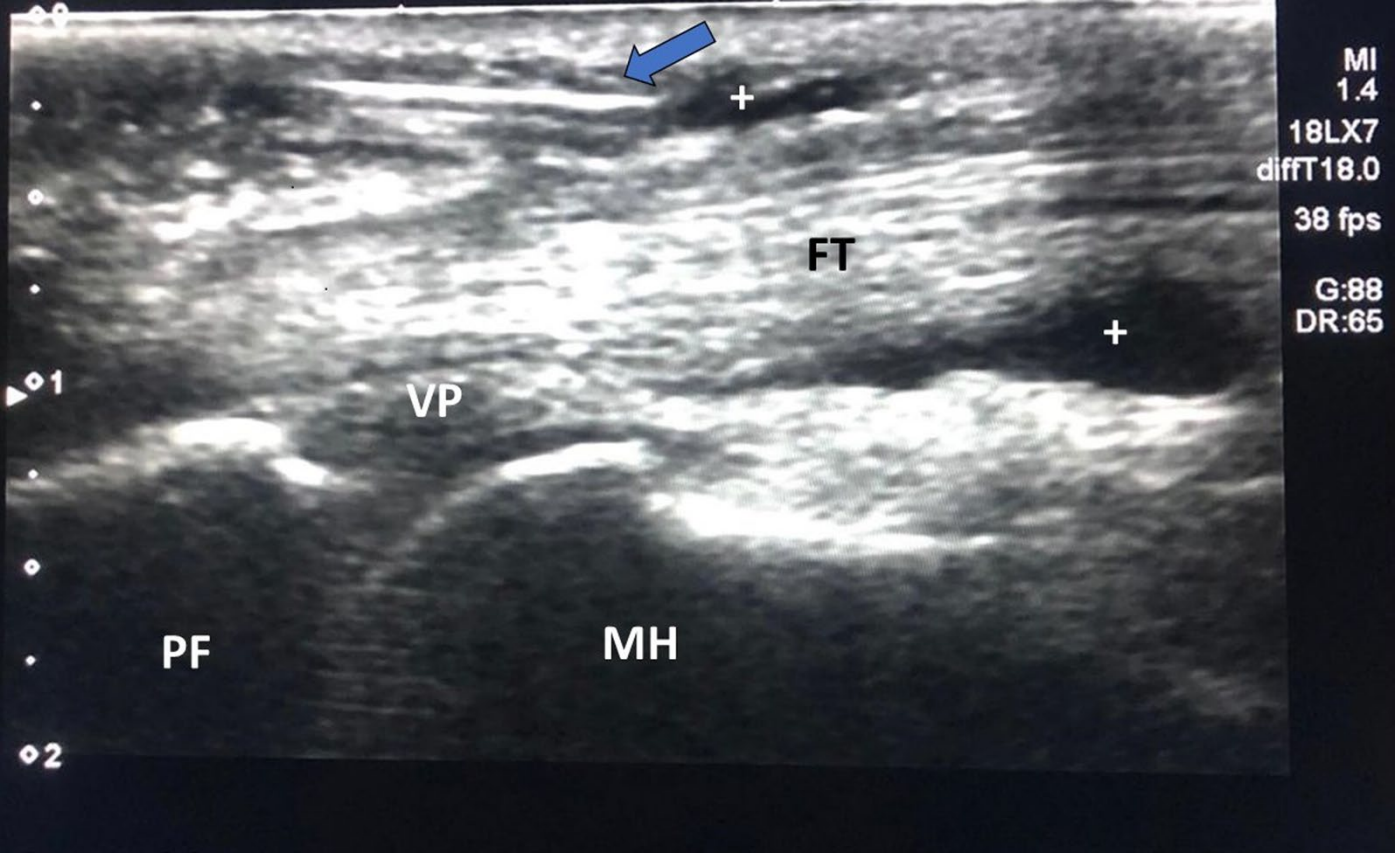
Ultrasound guided trigger finger injection. Arrow: 27 G needle, + : inside of the swollen flexor tendon sheath after injection, *FT* Flexor tendon, *VP* Volar plate, *PF* Proximal phalanx, *MH* Metacarpal head
